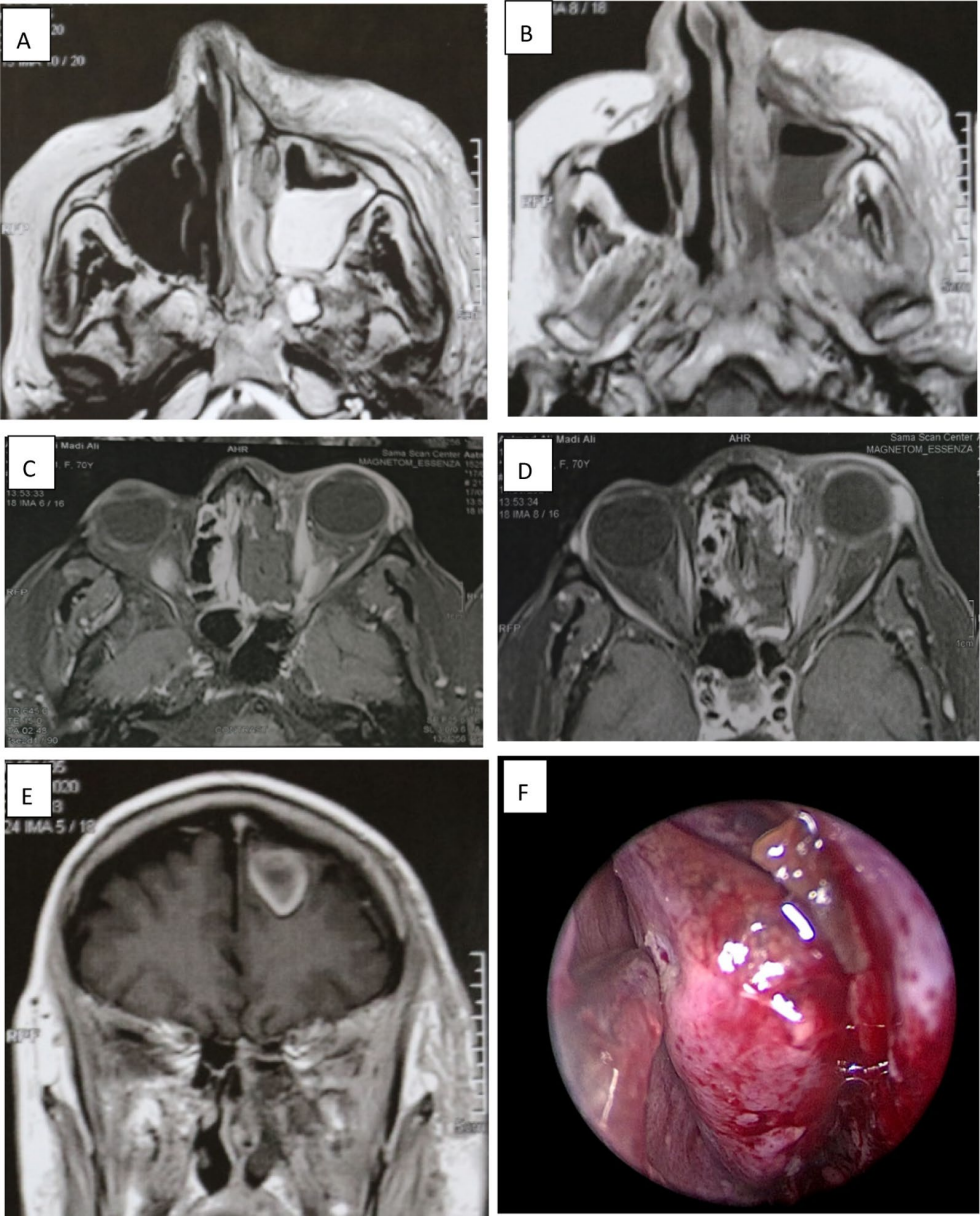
A 70-year-old female presented with left proptosis, visual loss, total ophthalmoplegia, severe headache and left facial gangrene after 4 weeks of COVID-19 infection with poor diabetic control. A MRI axial T2WI shows mucosal opacification of maxillary, left nasal cavity. B Contrast-enhanced T1WI shows enhanced mucosa with soft tissue thickening. C, D T2 with fat suppression shows mucosal thickening of the ethmoidal air cells, Infiltration medial orbital fat. E Contrast-enhanced T1 shows ring-enhanced brain lesion (brain abscess). F Endoscopic view shows necrosis and gangrene of the nasal turbinate and lateral nasal wall. Patient received treatment in the form of left maxillectomy, orbit exenteration, and abscess drainage and IV amphotericin B but patient died after 2 weeks of surgical treatment
